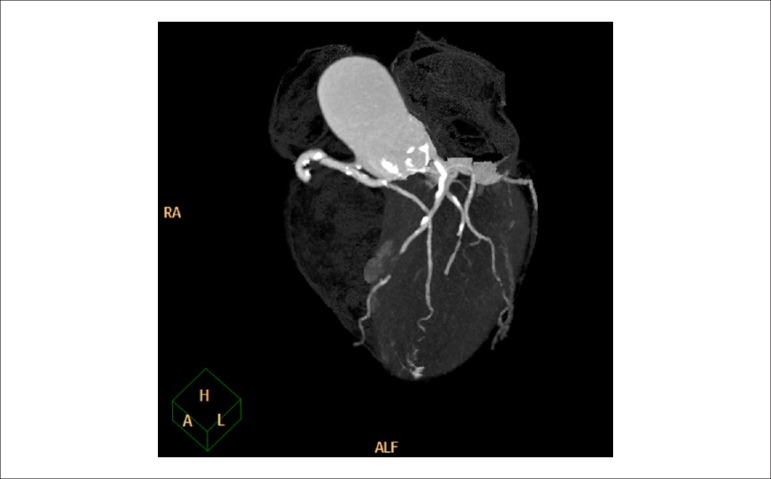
Case 10 - Coronary angio-CT showing significant coronary atherosclerosis, with multivascular calcification. Reproduced with the permission of Vitola JV.^234^
